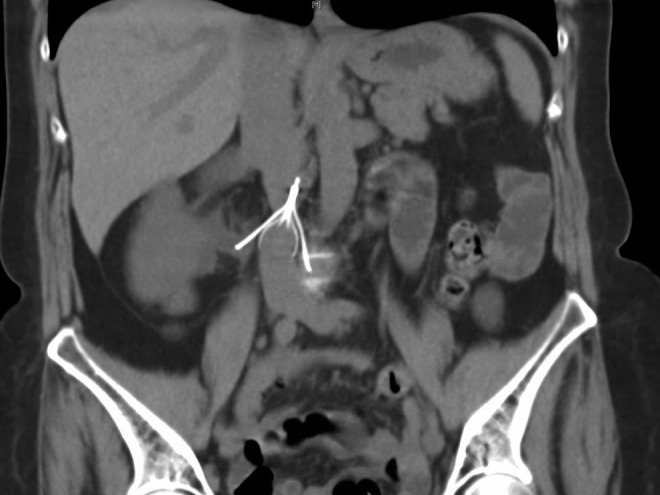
Coronal cut CT with additional demonstration of filter limb penetrating right ureter.
